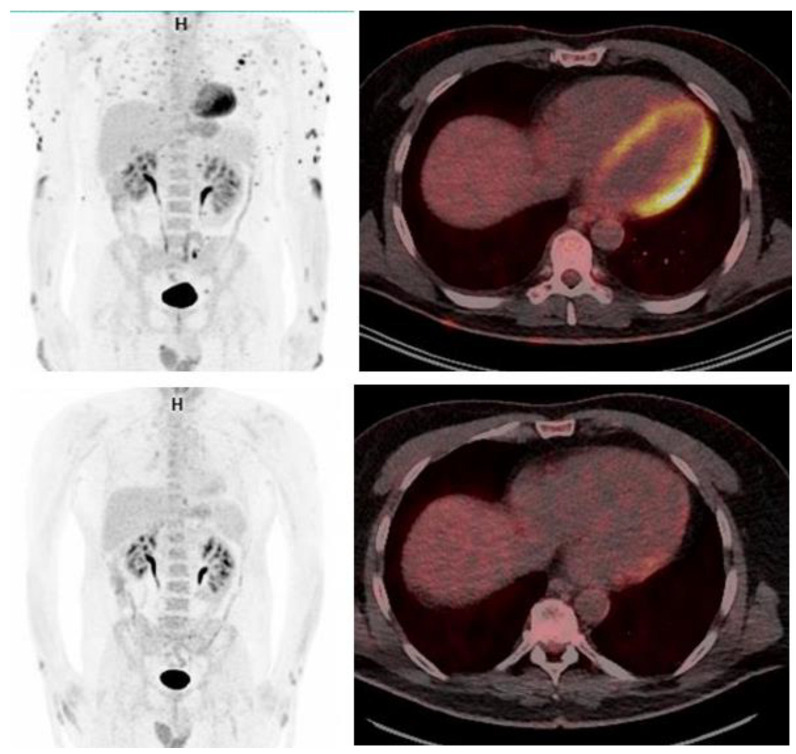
FDG PET scan before (upper panels) and after cobimetinib (lower panels), showing that most spots of increased metabolic activity, including the heart.
